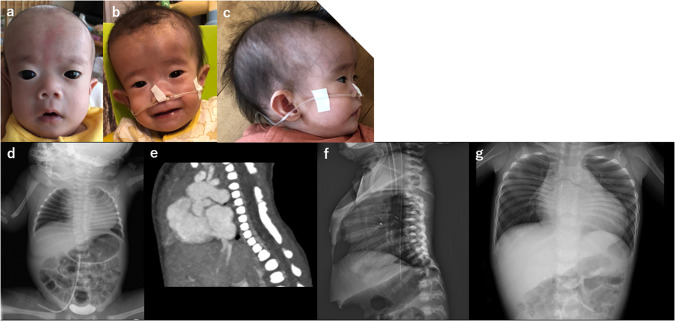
Clinical and radiological features. Facial appearance of the patient at six (a), 10 (b), and 11 months of age (c). Hypertelorism, a prominent forehead, full cheeks, low-set and posteriorly rotated ears, a broad nasal bridge, anteverted nares, and a long philtrum were noted. Chest radiographs taken at 1 d of age show cardiomegaly, thoracic vertebral hypoplasia, and thoracic hypoplasia (d). Computed tomography examination at 1 d of age and chest radiography at 19 days of age show extensive fusion at C6–T1 and T2–10 (e, f). Longitudinal growth of the thorax was also inhibited at 21 months of age (g).
